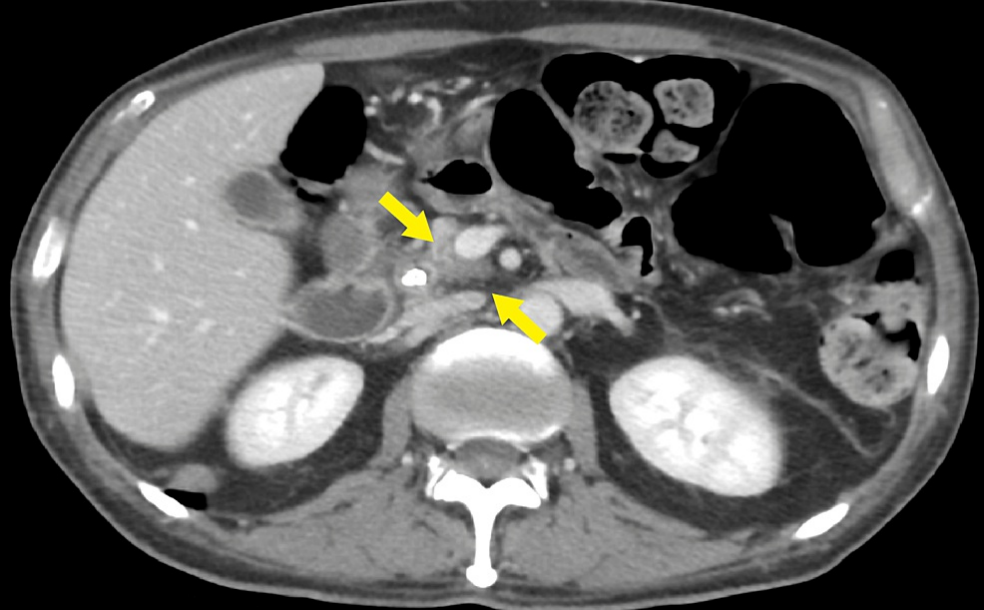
Post-neoadjuvant therapy axial CECT image shows significant improvement in the size of the pancreatic head adenocarcinoma There is persistent abutment of the portal vein/SMV confluence. The SMA is spared. CECT: contrast-enhanced computed tomography; SMV: superior mesenteric vein; SMA: superior mesenteric artery
